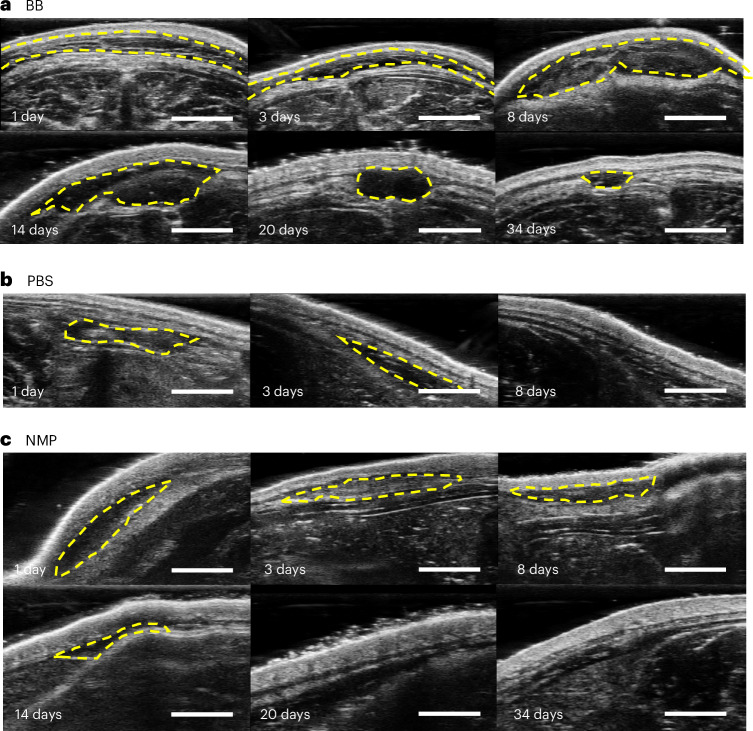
Assessment of solvent exchange in vivo*.* a–c, Ultrasound images depicting the clearance of BB within subcutaneous tissue over 34 days (a), PBS clearance over 8 days (b) and NMP clearance over 20 days (c). Yellow dashed circles indicate the contour of the injected solvent. Scale bars, 5 mm.
